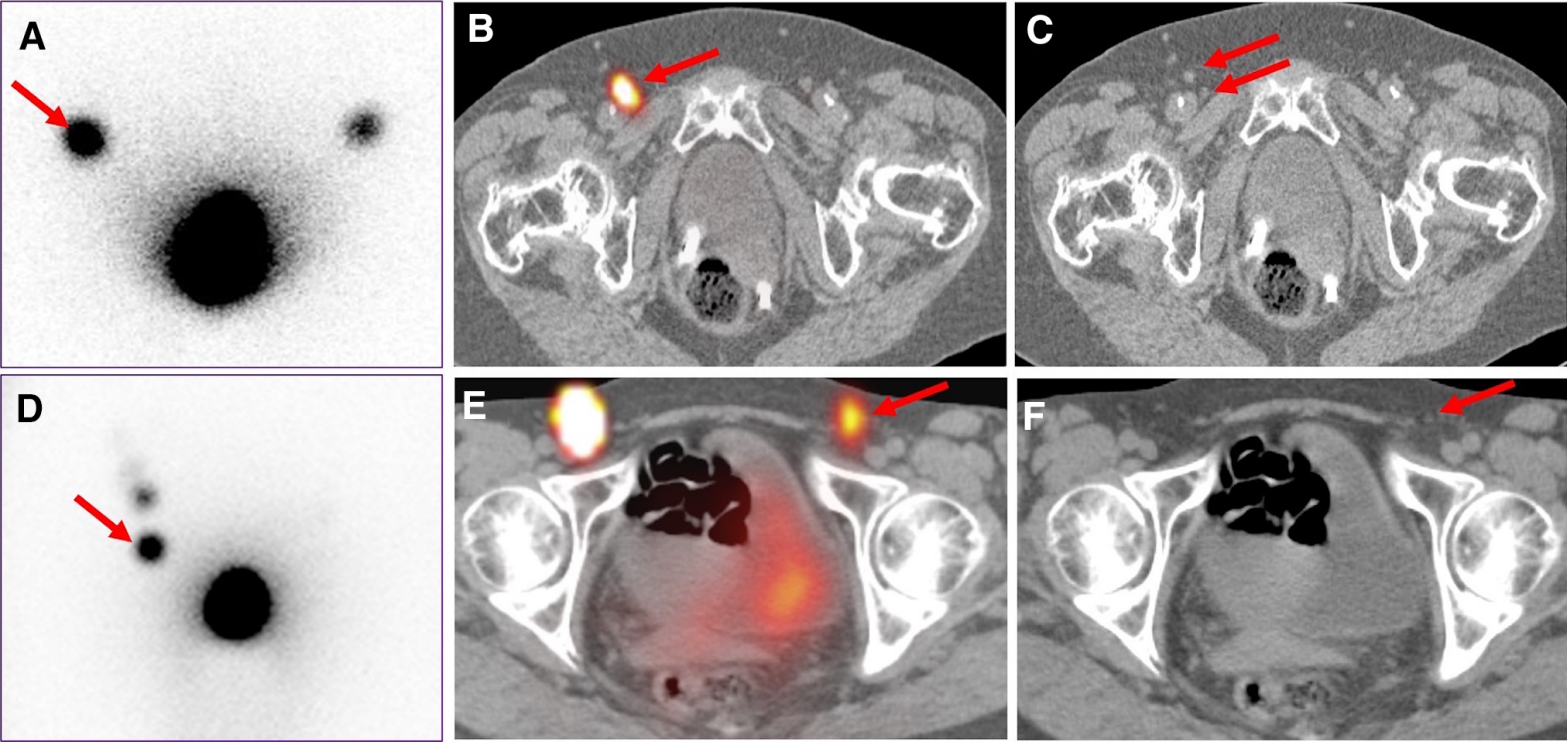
In a patient with vulvar cancer, delayed planar imaging (a) shows one SLN in the right groin (*red arrow*) corresponding with one allocated SLN uptake (*red arrow*) on transversal-fused SPECT/CT (b) and two not enlarged lymph nodes on transversal CT (c) (*double arrows*). In another patient, delayed planar image (d) shows unilateral lymphatic drainage with a single SLN in the right groin (*red arrow*), while transversal-fused SPECT/CT (e) shows bilateral drainage with also a contralateral SLN (*red arrow*) corresponding with a not enlarged lymph node in the left groin on CT (f)
